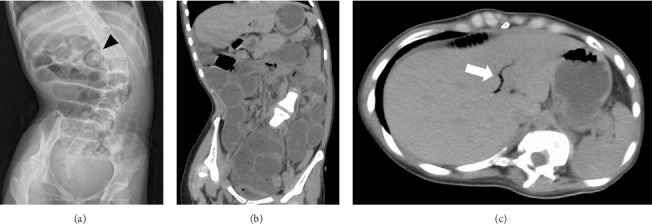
Plain radiograph and computed tomography (CT) of the abdomen in a patient with gallstone ileus. Abdominal radiograph demonstrates dilated small bowel (a). A gastrosomy button is indicated by arrowhead. Coronal (b) and axial (c) CT images demonstrate the small bowel obstruction and pneumobilia (arrow), respectively.
